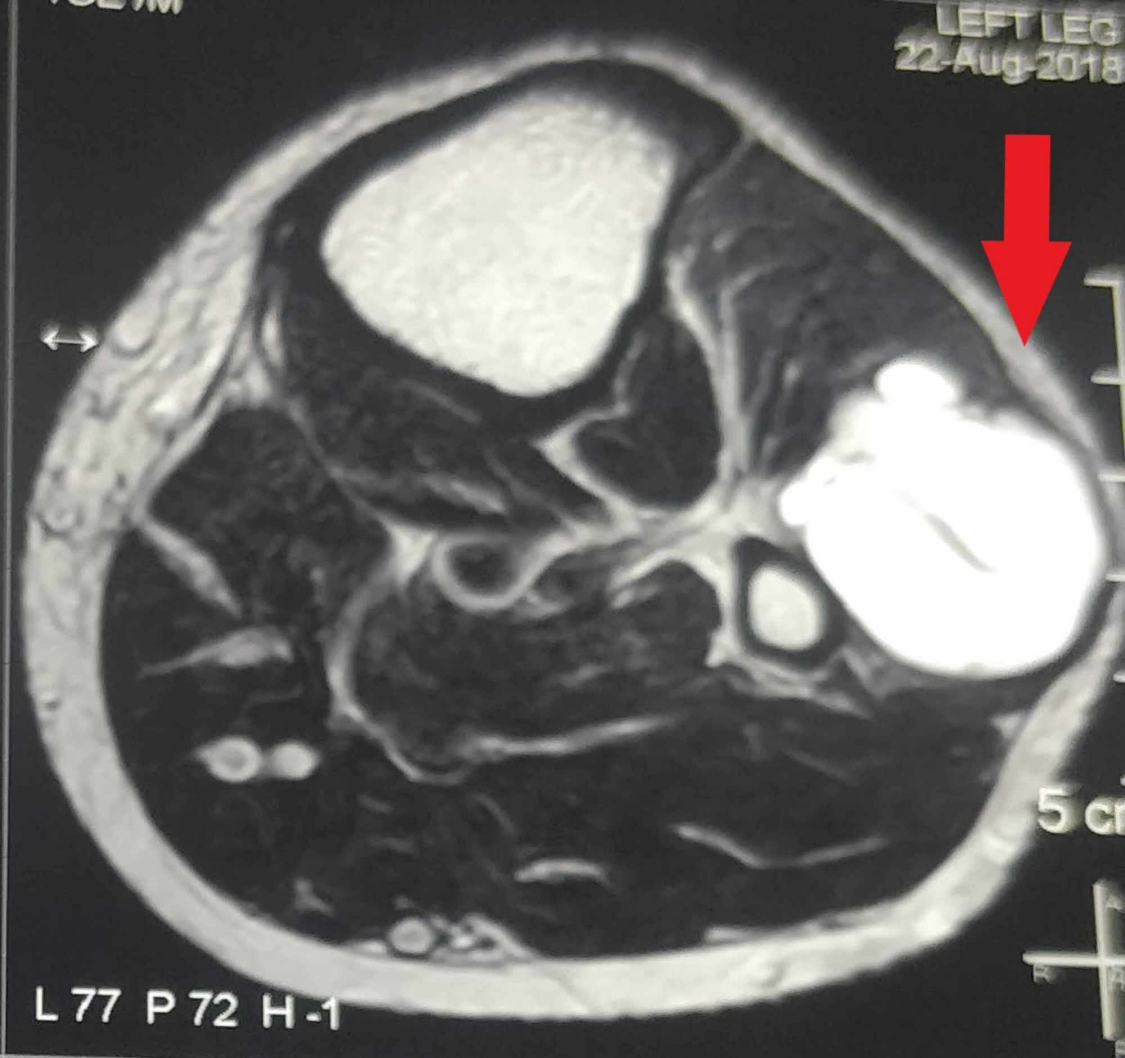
Axial cut of magnetic resonance imaging showing ganglion cyst (pointed by the red arrow)
